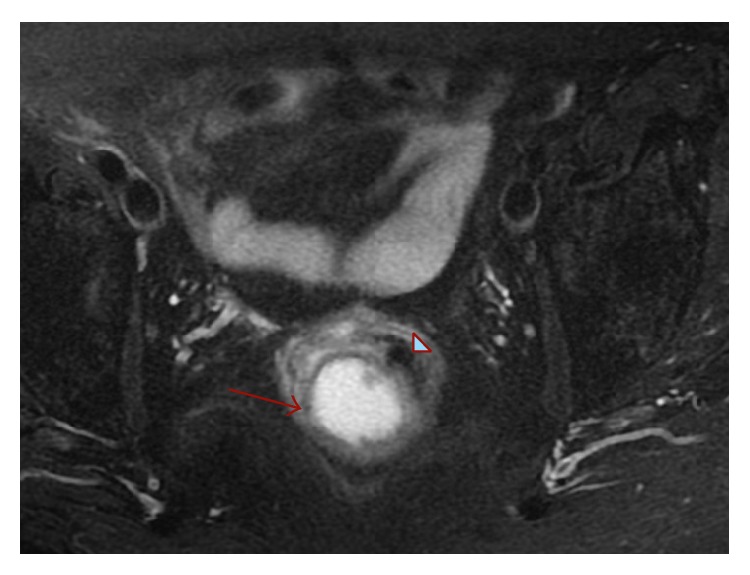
Pelvic MRI. Mass (red arrow) compressing intestinal lumen.
